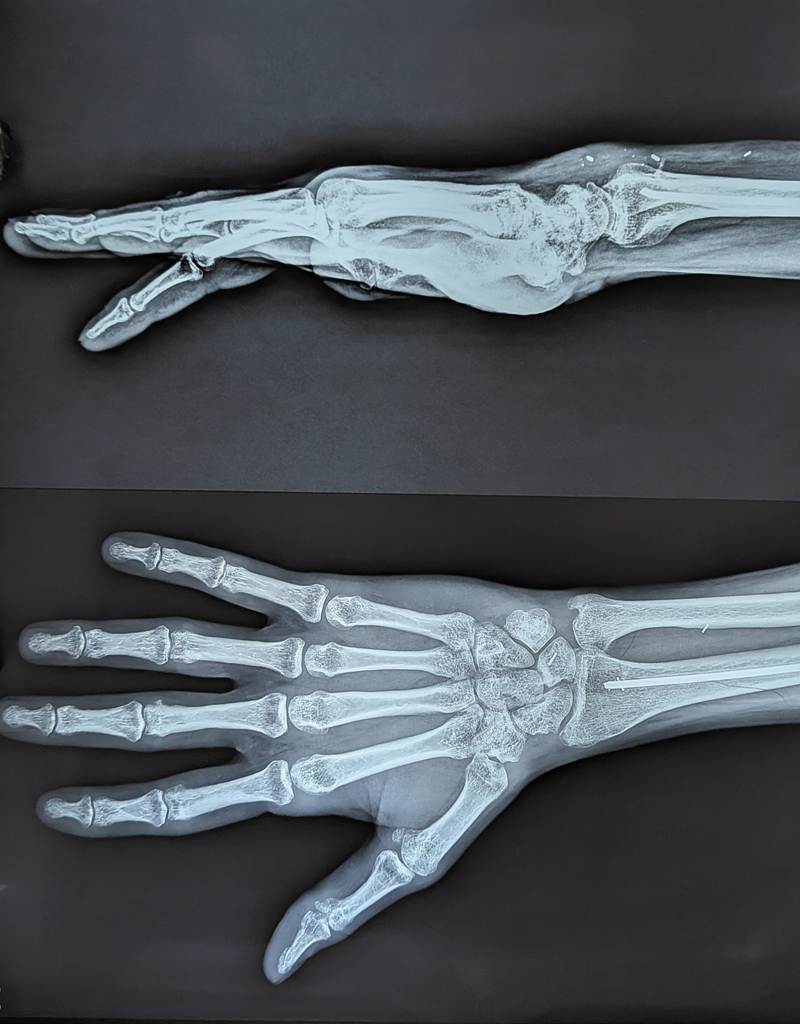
Postoperative radiograph of the right hand and distal forearm showing the shortened intramedullary nail no longer impinging the extensor compartment.
